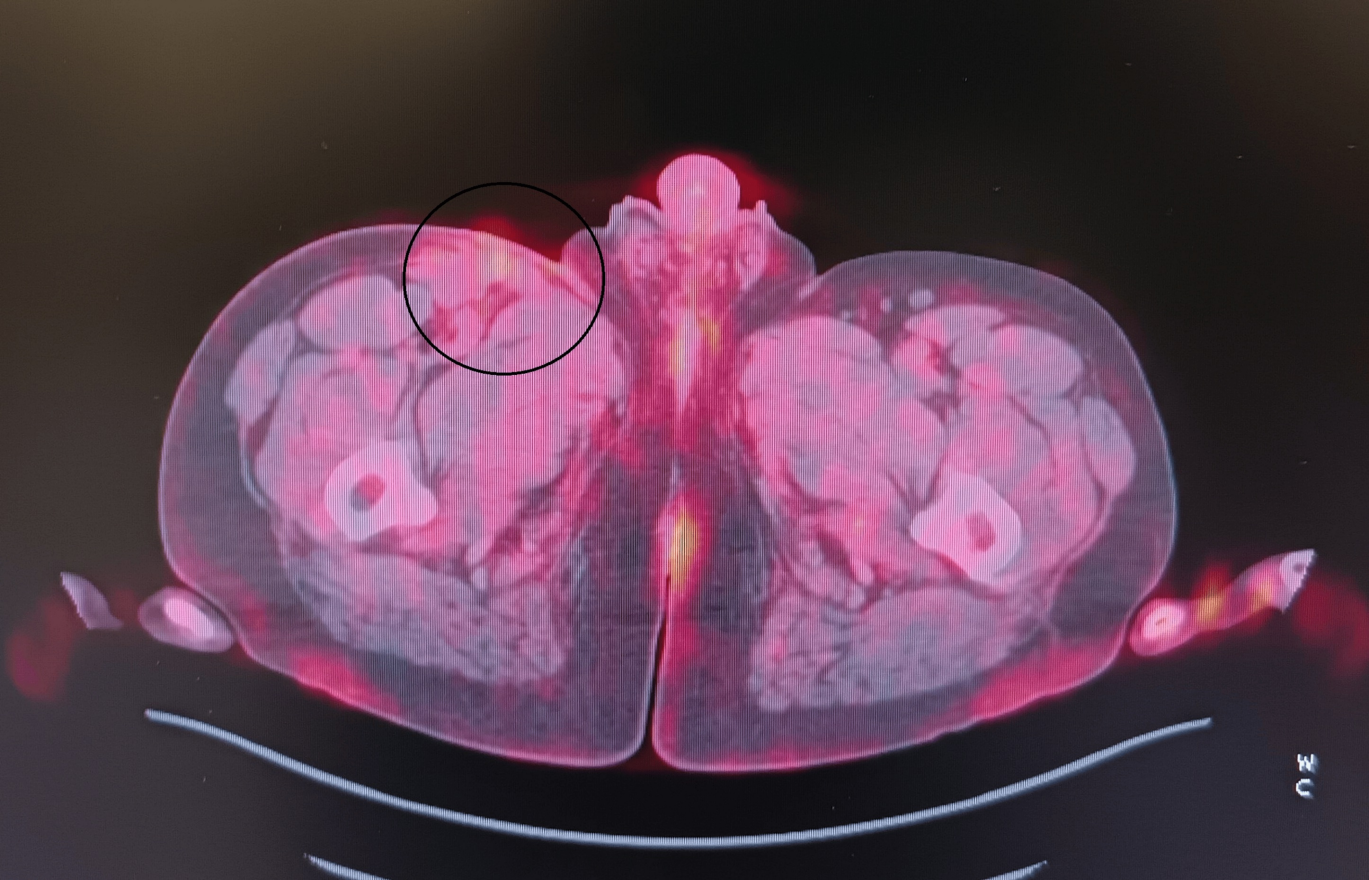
Increasing FDG uptake in the right iliac region, suggested early metabolic progression (SUVmax = 11.64). FGD: fludeoxyglucose; SUVmax: maximum standard unit value
